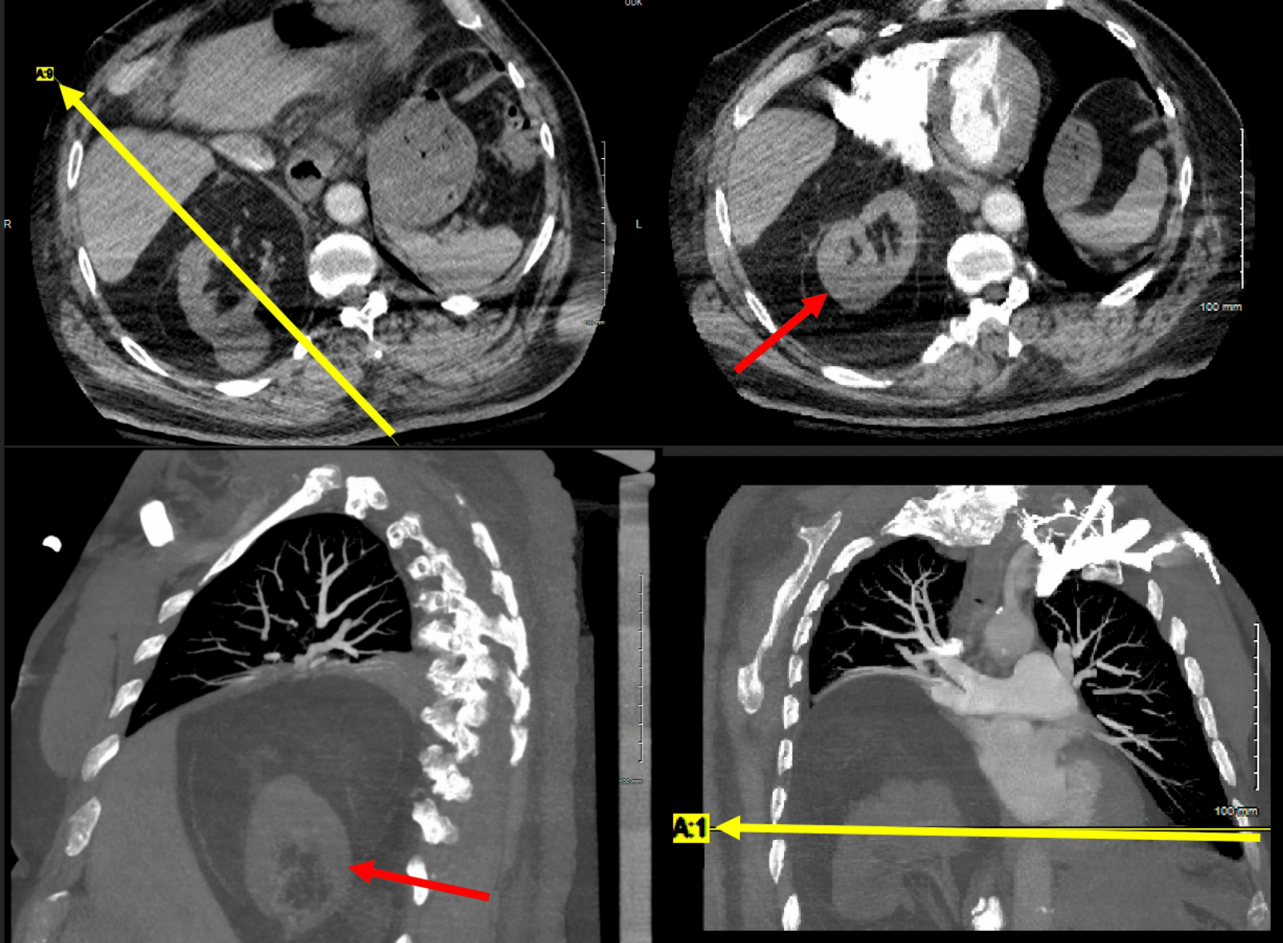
Chest CTA demonstrating marked elevation of the right hemidiaphragm with retroperitoneal fat and the right kidney located within the thorax Top left: Axial CT scan, with a yellow arrow line indicating the plane of the corresponding sagittal view (bottom left). Bottom left: Sagittal CT view, with a red arrow marking the ectopic kidney. Top right: Axial CT scan, with a red arrow marking the ectopic kidney. Bottom right: Coronal CT view, with a yellow arrow line indicating the image plane from the axial scan above (top right).
